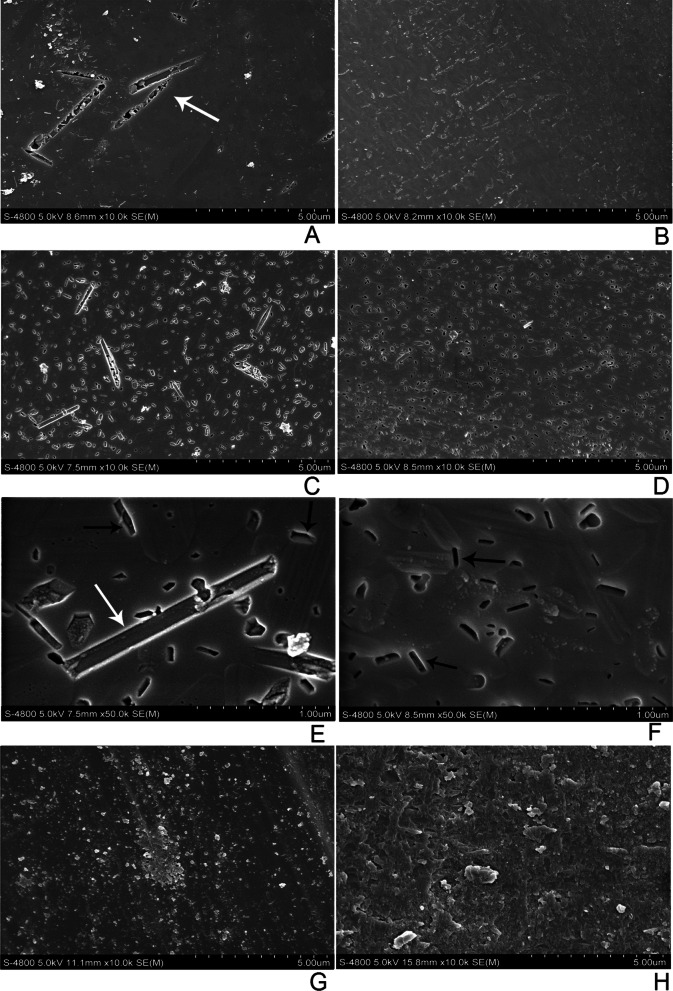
SEM images under high magnification of the as-worn surfaces developed in different environmental conditions: LD in a wet environment without ultrasonic cleaning (A) and after removal of the tribofilm with ultrasonic cleaning as revealed in C and E; LD in a dry environment without ultrasonic cleaning (B) and after ultrasonic cleaning (D) and (F); LEU in a wet environment (G); LEU in a dry environment (H); the white arrow indicates needle-like hole; the black arrow indicates the small micropores along the edge of LD crystals
